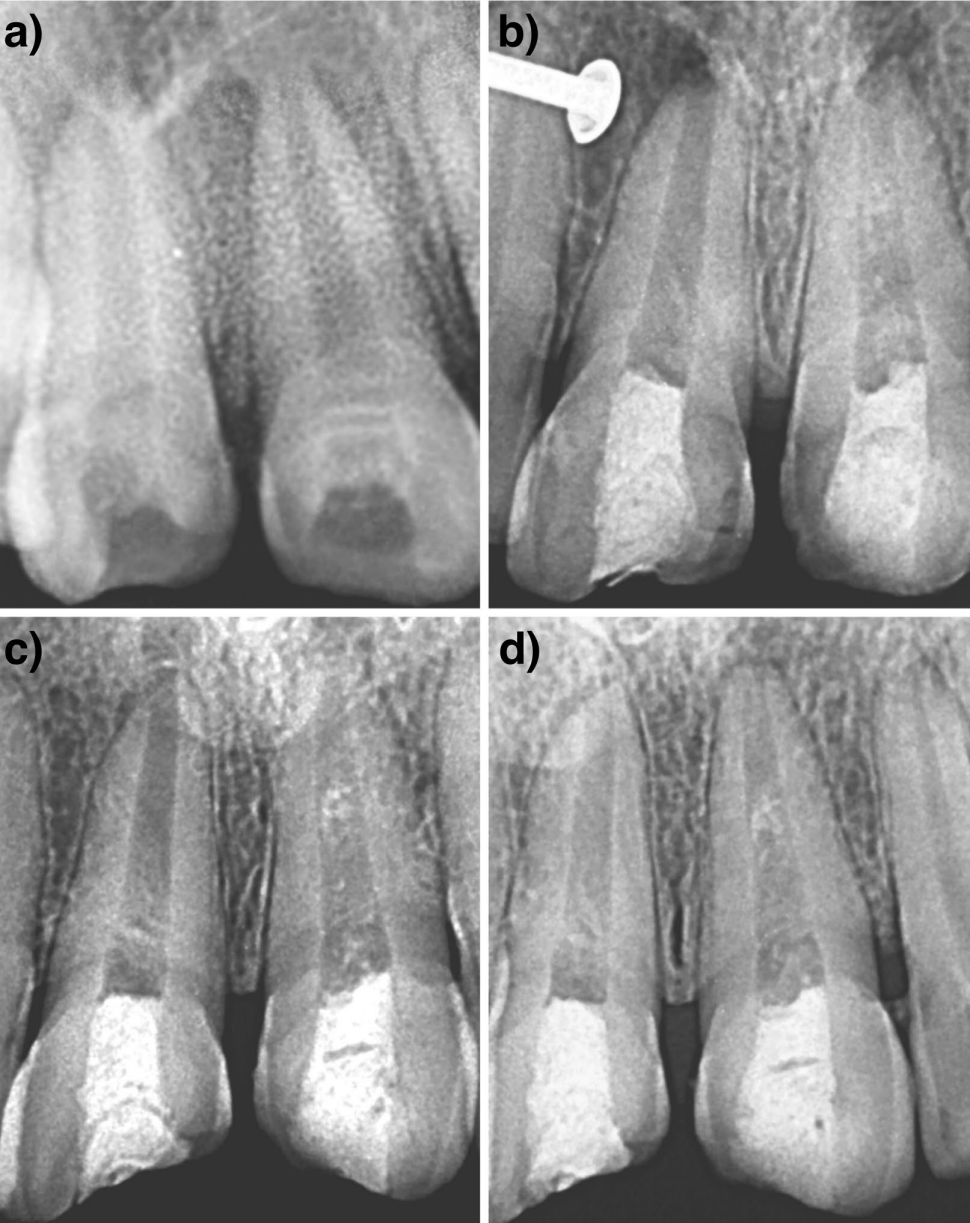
Case 1: Tooth no. 11, 21- (A) Preoperative radiograph; (B–D) Radiographs at 6 months, 12 months and 24 months follow-up period.
